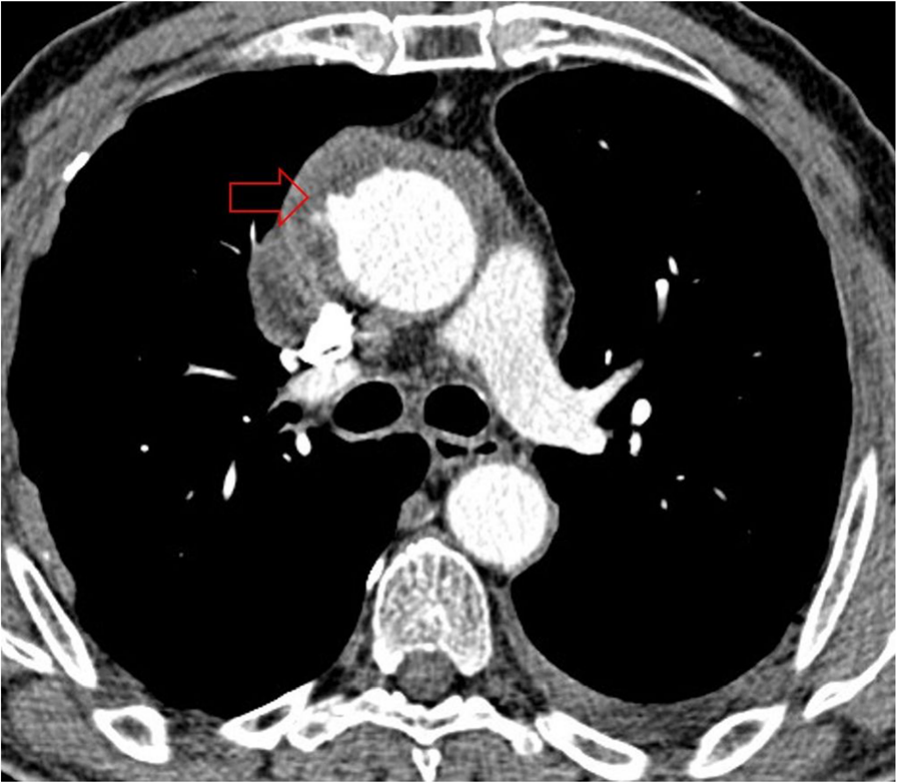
Axial chest CT image in the mediastinal window shows pseudoaneurysm formation and wall erosion in ascending aorta (red arrow)
